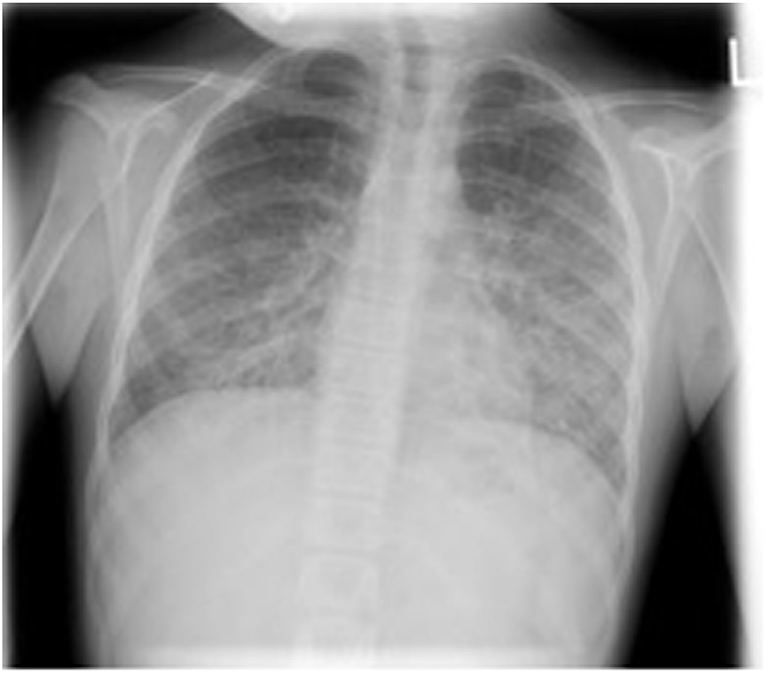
Chest X-ray demonstrating bilateral pulmonary infiltrates caused by pneumocystis pneumonia.
